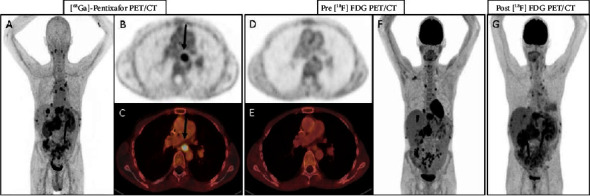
(A–C): [68Ga]Pentixafor PET/CT, (D–F), [18F]FDG PET/CT before application and (G) after application of therapeutic CXCR4 ligand, 2 GBq [90Y]Pentixather of patient 1.
